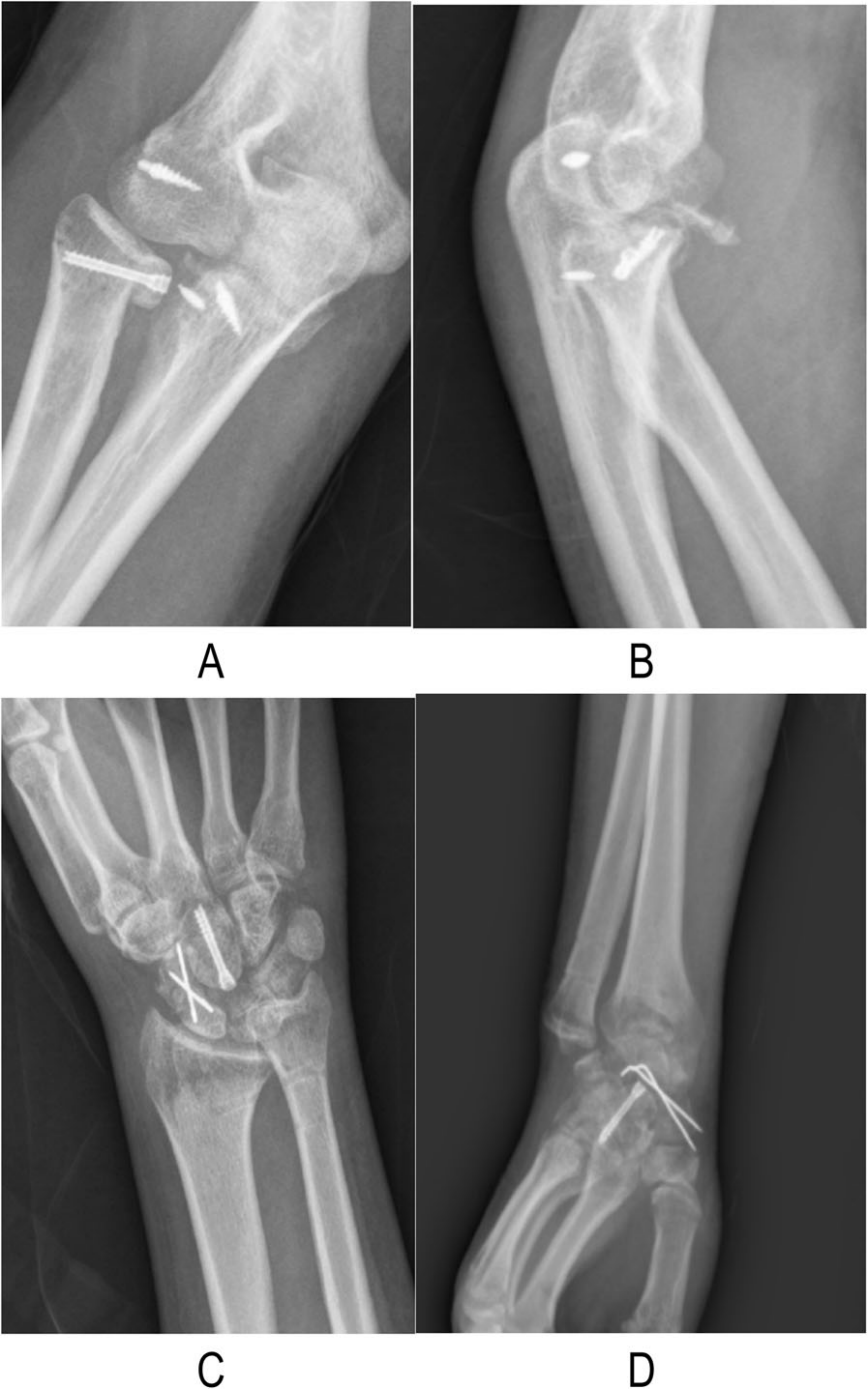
Two months after first surgery radiographs. a-b, elbow joint; c-d,wrist joint
